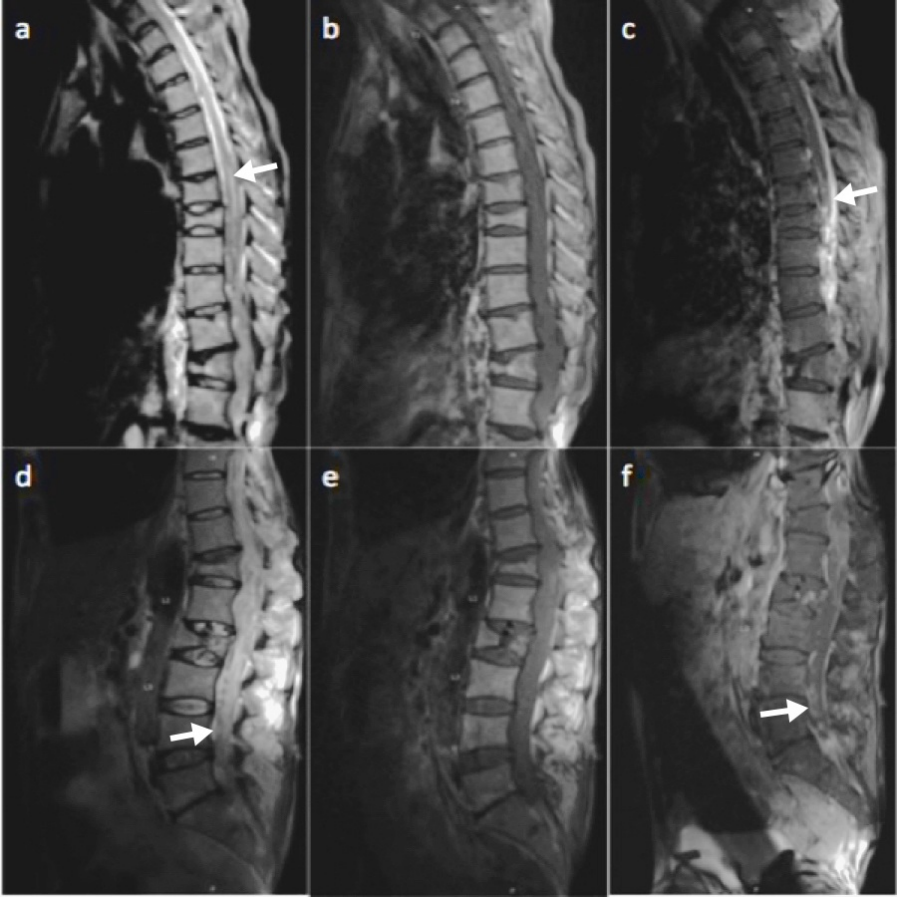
MRI scan of thoracic (upper row) and lumbar spine (lower row) with and without contrast [a] T2W shows a diffuse hyperintensity (arrow) involving the central portion of the thoracic spinal cord. [b] T1W C- shows a diffuse low signal involving the thoracic spinal cord. [c] T1W C+ shows a diffuse dural and pial enhancement (arrow) with no definitive focal fluid collections. [d] T2W shows a heterogeneous signal throughout the lumbar spinal canal (arrow) with areas of multiloculated T2 hyperintensity. [e] T1W C- shows a diffuse low signal involving the cauda equina. [f] T1W C+ shows a multiloculated enhancement of the cauda equina (arrow) and the peripheral margins of the lumbar spinal canal.
